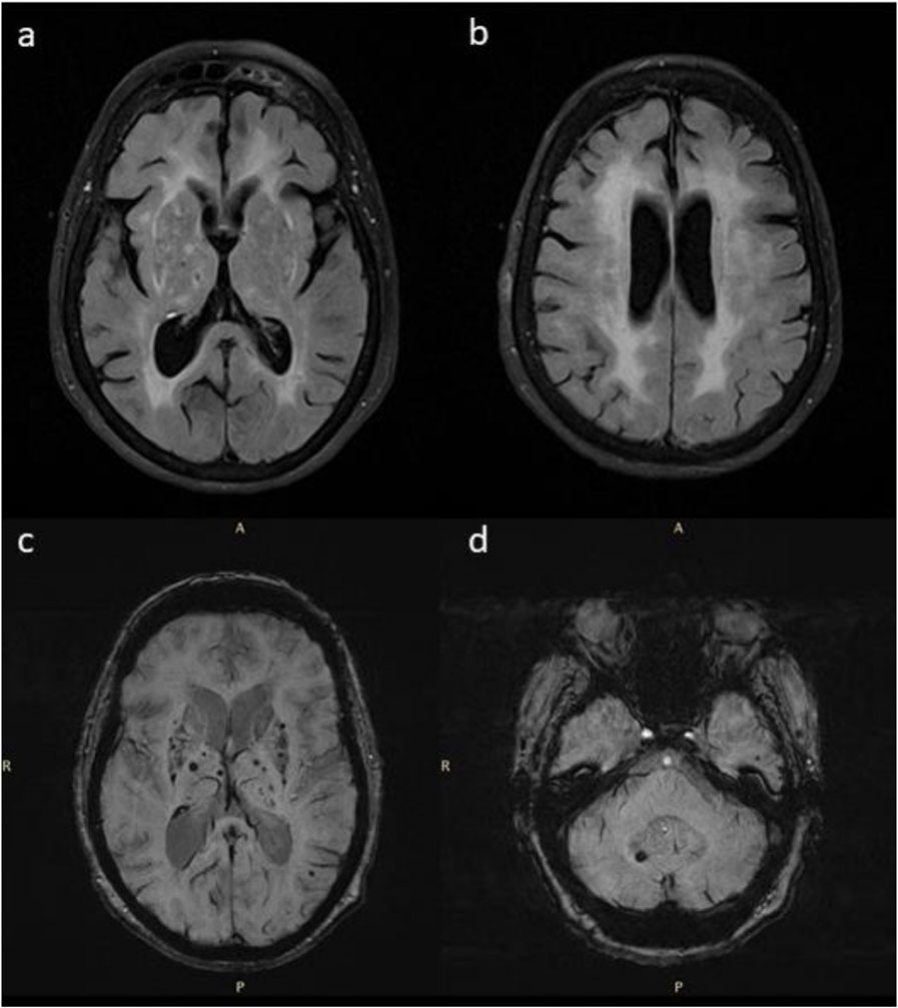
brain MRI scans: a-b) axial FLAIR sequences, showing diffuse white matter hyperintensity, including external capsules. C-d) Susceptibility-weighted imaging (SWI) showing hemosiderin-depositions in the basal ganglia (thalami included) and in the right cerebellum
